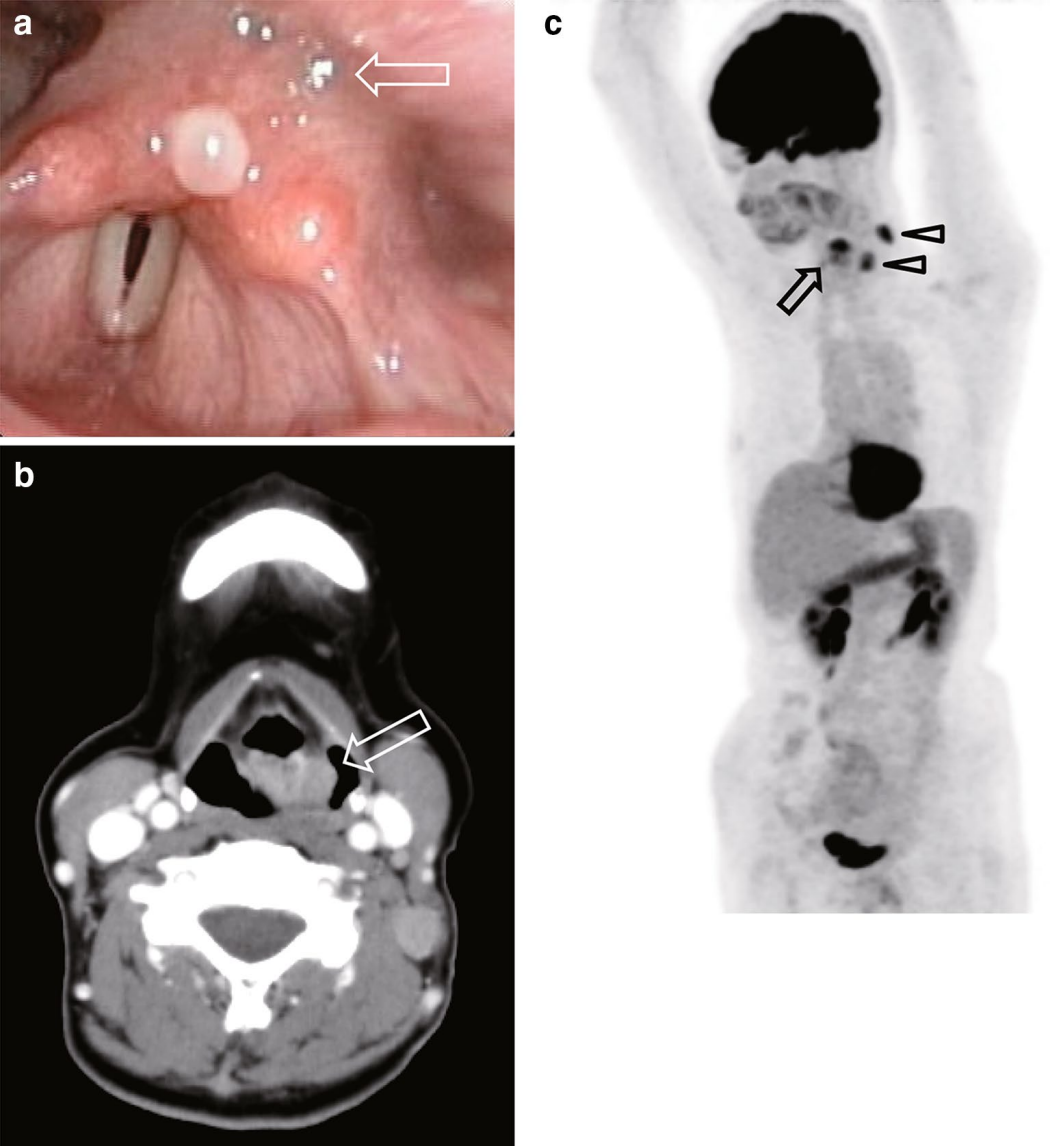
a On a hypopharyngeal fiberscopic image, a tumor was observed in the postcricoid area (a *white arrow*). b On a contrast-enhanced computed tomography scan of the neck before treatment, an enhanced tumor was observed in the postcricoid area of the hypopharynx (a *white arrow*). c On a fluorodeoxyglucose positron emission tomography scan of the neck before treatment, an enhanced tumor was observed in the hypopharynx (a *black arrow*) and the left side of the neck (*arrowhead*)
